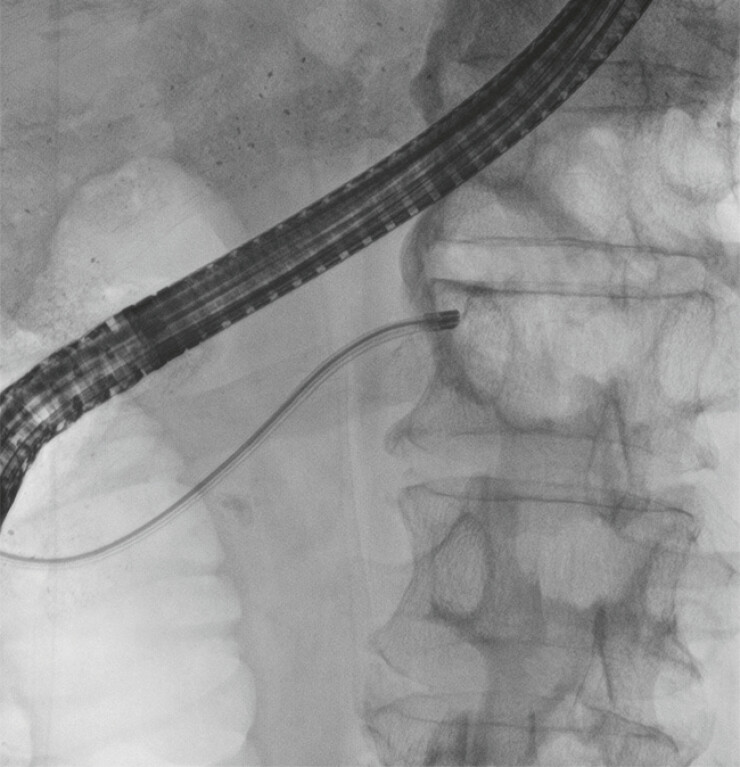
Radiographic images of POPS being advanced toward the pancreatic duct obstruction via the minor papilla. POPS, peroral pancreatoscopy.
